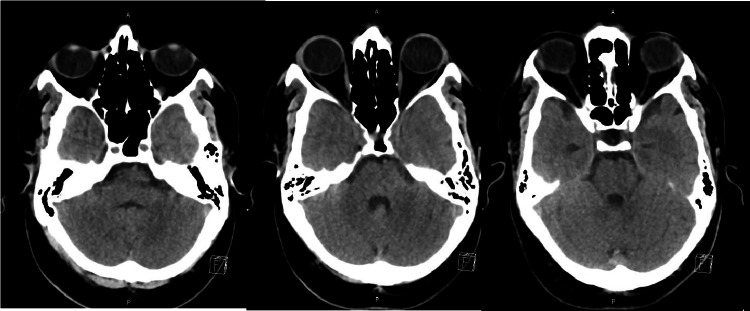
Axial noncontrast CT head of the patient at the level of pons/posterior fossa (multiple sections) showing no abnormalities. CT: computed tomography
